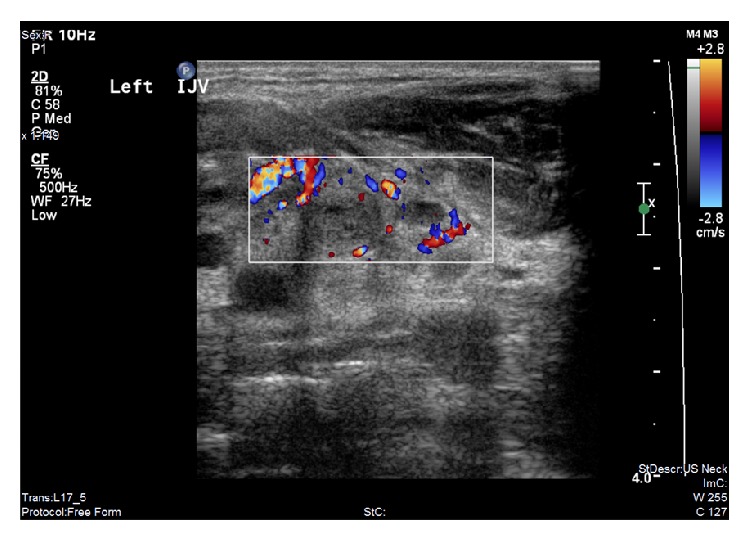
Doppler ultrasound of the neck showing the thrombus in the internal jugular vein.
